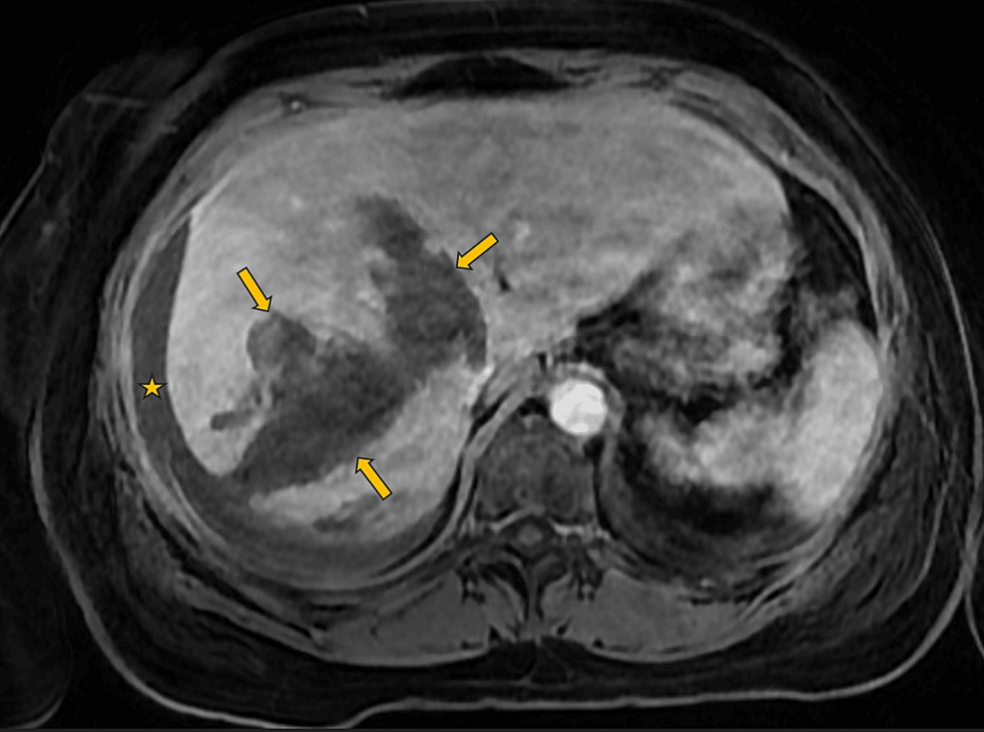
Hospital day 7: MRI abdomen and pelvis T1 phase Arrows - identify the edges of the hepatic laceration/hematoma; Star - extrahepatic hematoma
